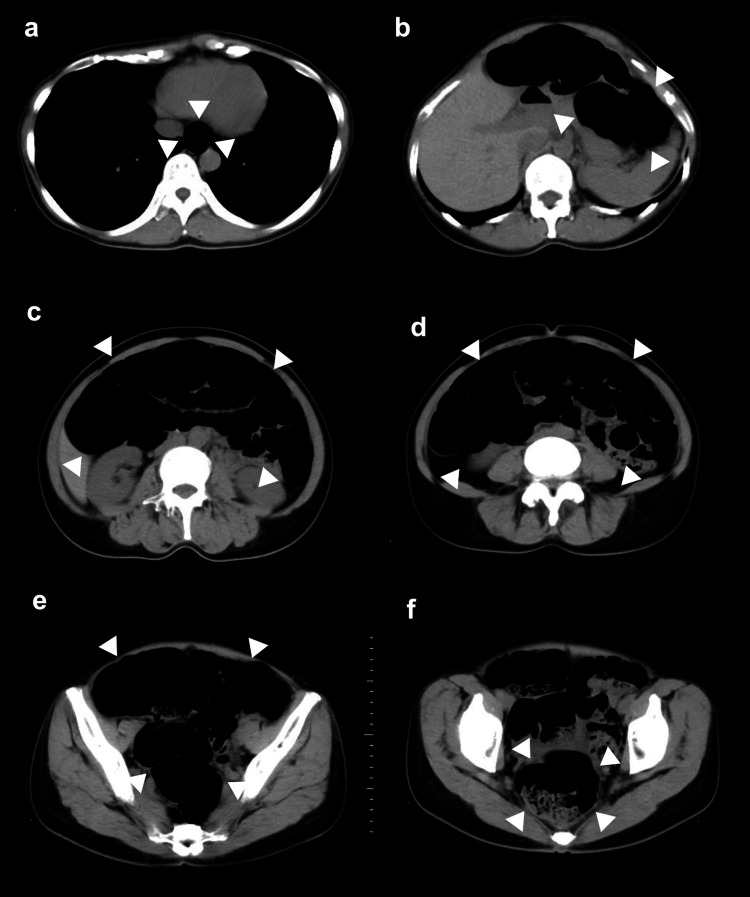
Computed tomography Computed tomography showed no stenosis of the digestive tract and continuous gas filling from the esophagus (Figure 2a, arrow) to the stomach (Figure 2b, arrow), from the small intestine to the colon (Figures 2c, 2d, 2e) and rectum (Figure 2f, arrow).
